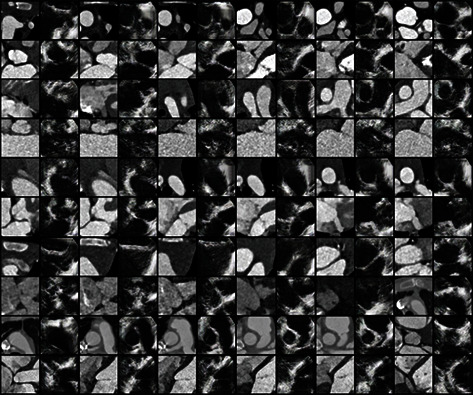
The TEE-like images generated from CT images.
